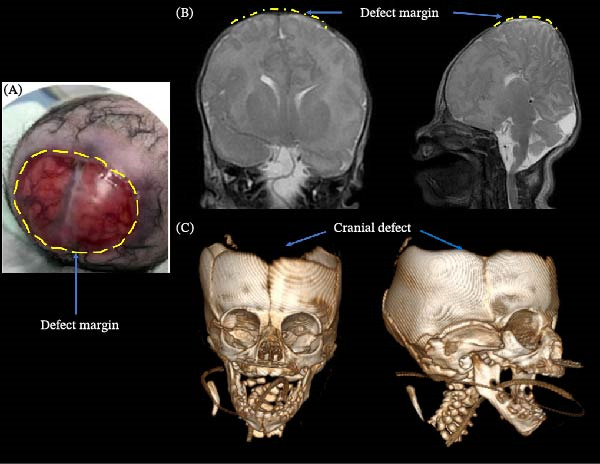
Patient’s preoperative condition. (A) clinical picture of a large defect of aplasia cutis congenita involving the skull and dura mater. (B) Head MRI reveals normal intracranial content without hydrocephalus or other congenital anomalies. (C) Head CT‐scan reveals a large skull defect involving the parietal bones.
